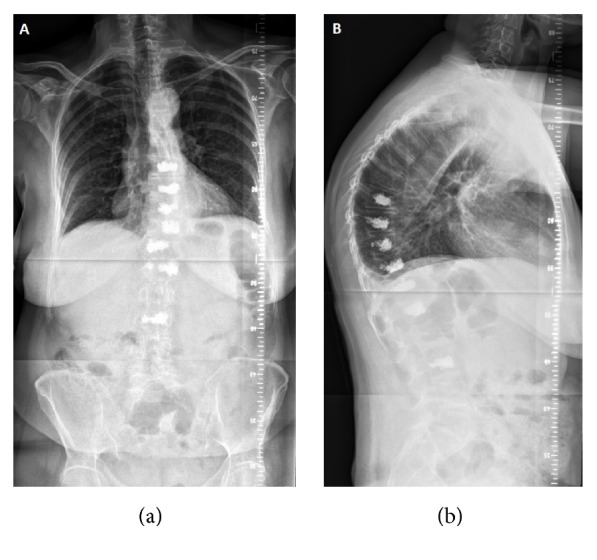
Postoperative anteroposterior (a) and lateral (b) radiographs of the spine.
